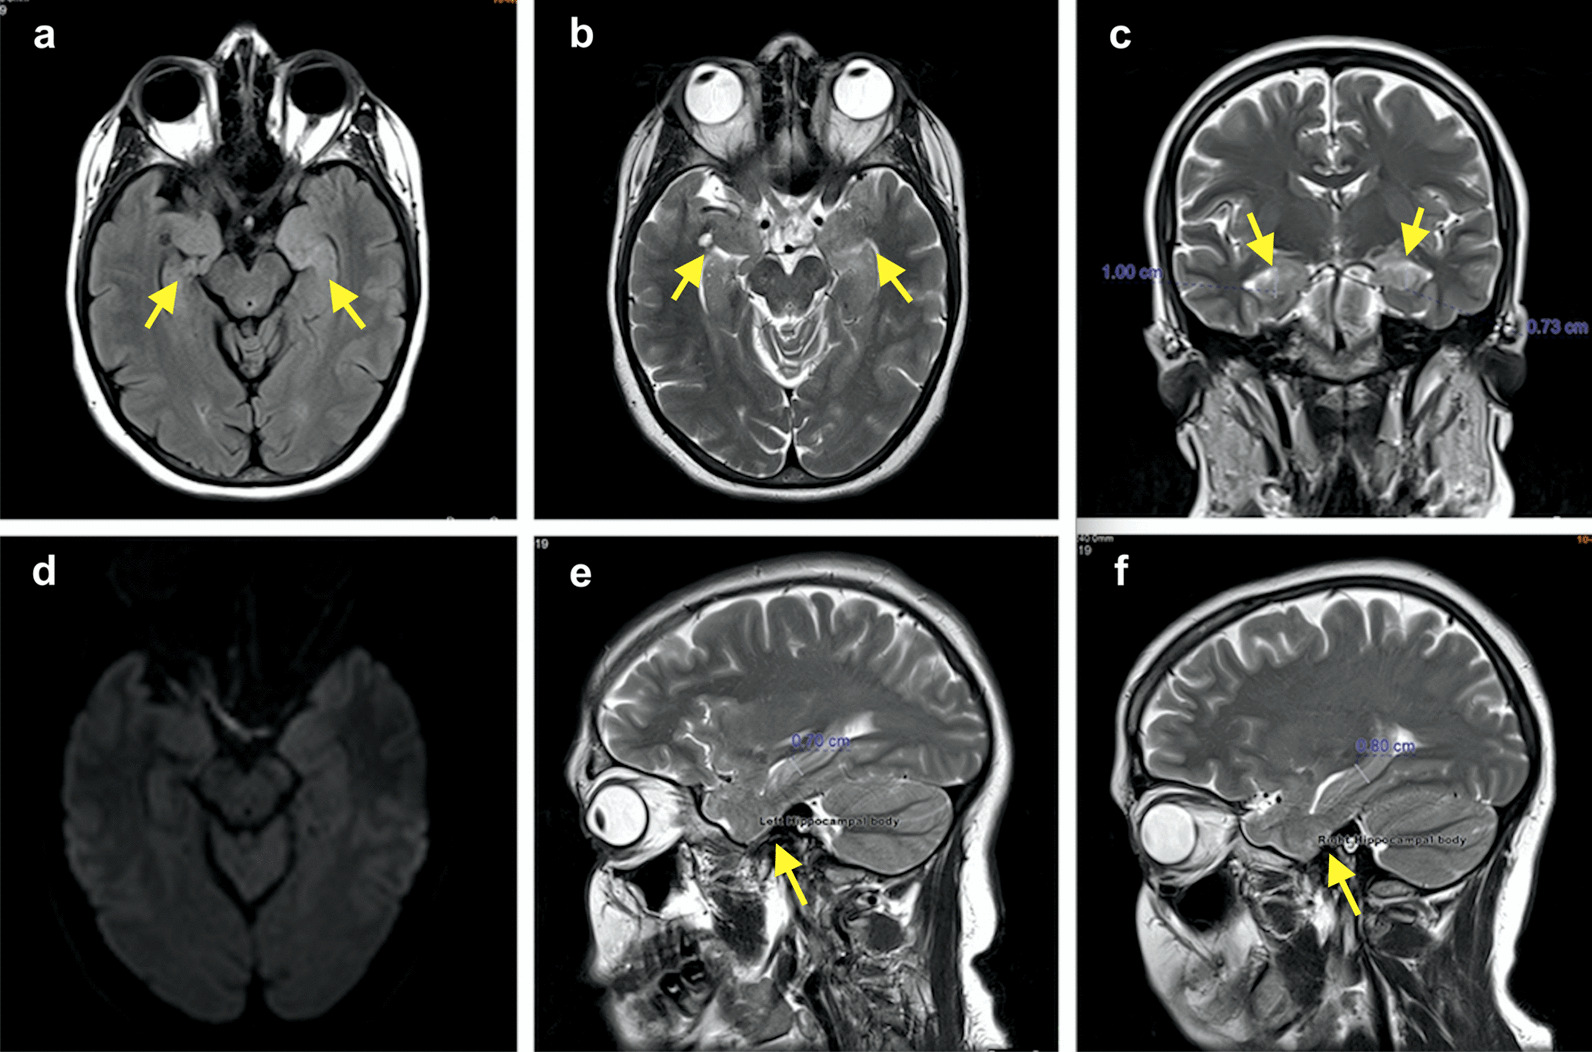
Follow-up magnetic resonance imaging after 2 months of immunotherapy. a Axial fluid-attenuated inversion recovery (FLAIR), b axial T2-weighted images, c coronal T2-weighted images, e, f sagittal T2-weighted images, showing decrease in the T2/FLAIR high signal intensity of the hippocampi bilaterally, and regression of the hypertrophy of hippocampi (left: head thickness 7 mm, body thickness 8.5 mm, right: head thickness 10 mm, body thickness 8 mm) and amygdala bilaterally (arrows), d diffusion-weighted imaging showing no diffusion restriction.
